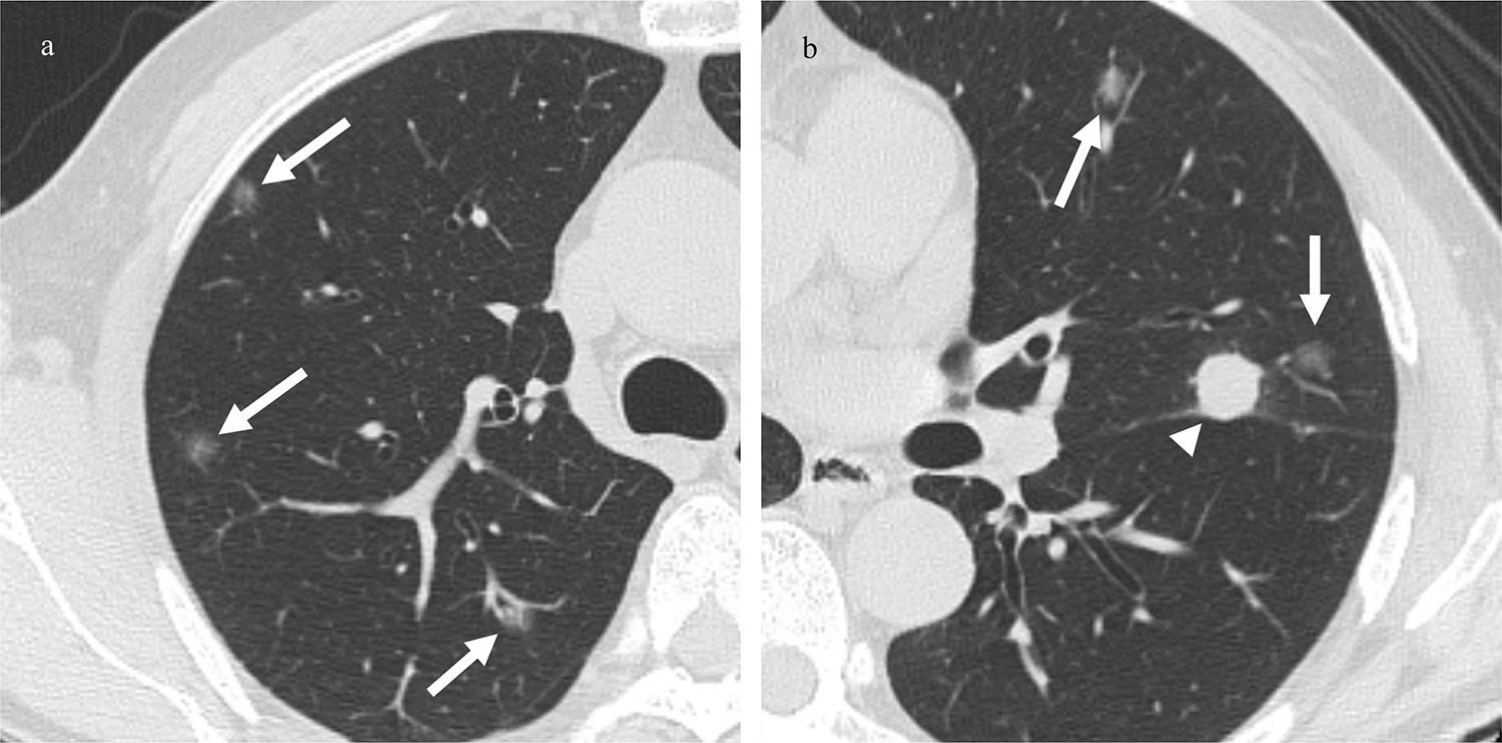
A 56-year-old man with mucosal melanoma with a GGN-dominant pattern. a, b. Follow-up CT 10 months after the operation shows multiple metastases as GGNs in bilateral lungs (white arrows). b. Some metastases appeared as solid nodules (white arrowhead). GGN, ground-glass nodule; CT, computed tomography
